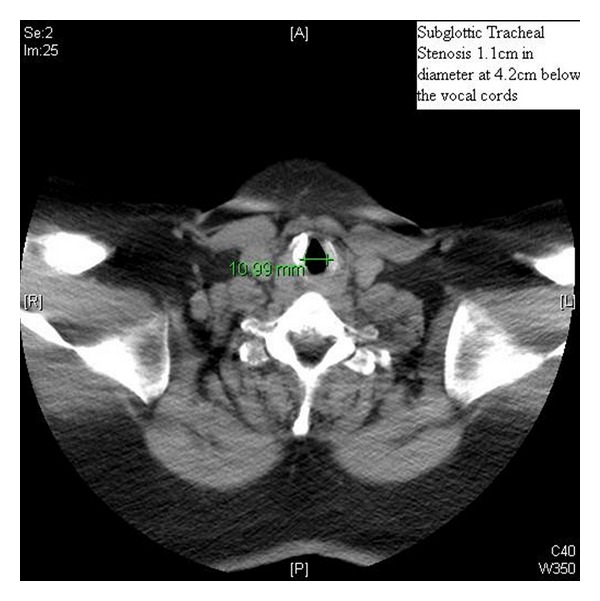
CT SCAN of subglottic tracheal stenosis.
